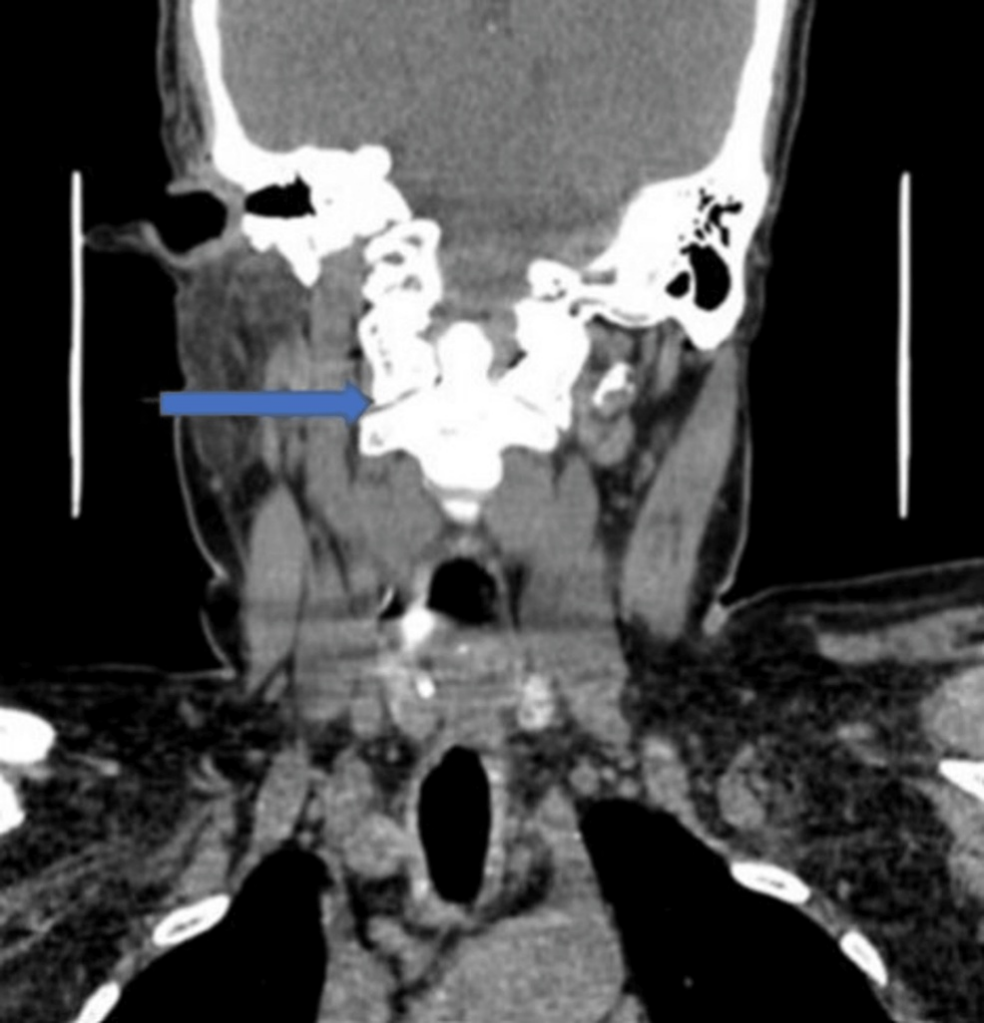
CT neck showing subglottic stenosis.
